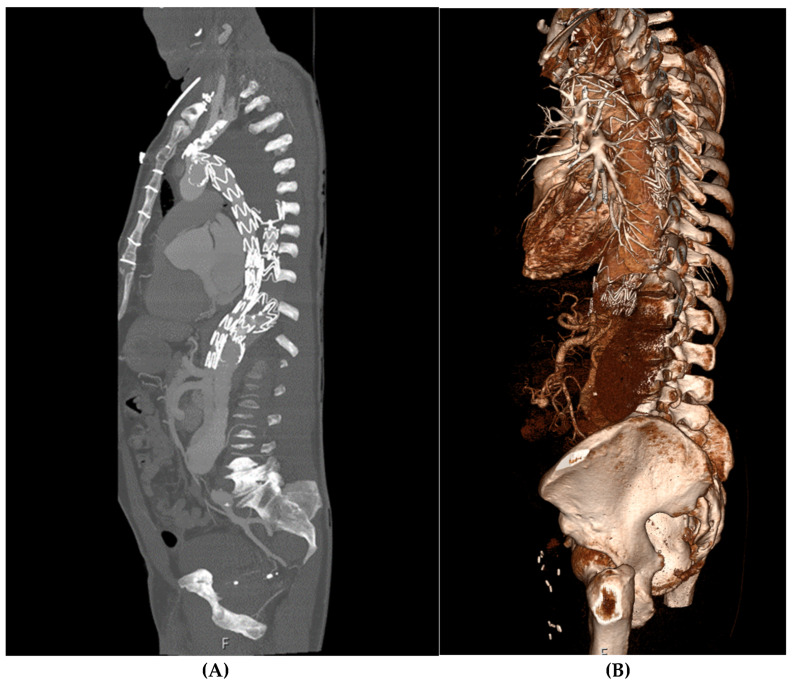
Postoperative computed tomography angiography (A) and three-dimensional rendering (B) of a patient treated with double candy-plug.
